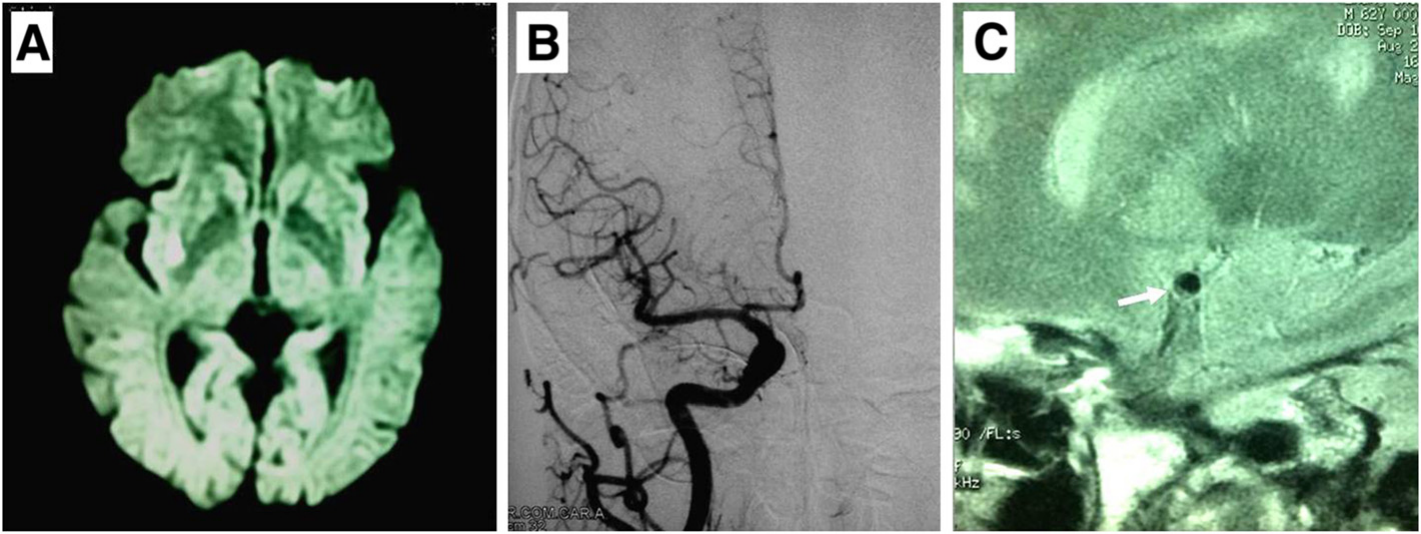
MRI, DSA and HR MRI findings of Case 1. (A) MRI diffusion weighted image shows a hypersensitive lesion on right putamen with rostral extension to the corona radiates. (B) Digital angiography of the right MCA shows normal. (C) High-resolution MRI of the MCA demonstrates an atherosclerotic plaque (arrow) on the frontal wall of the right proximal MCA.
